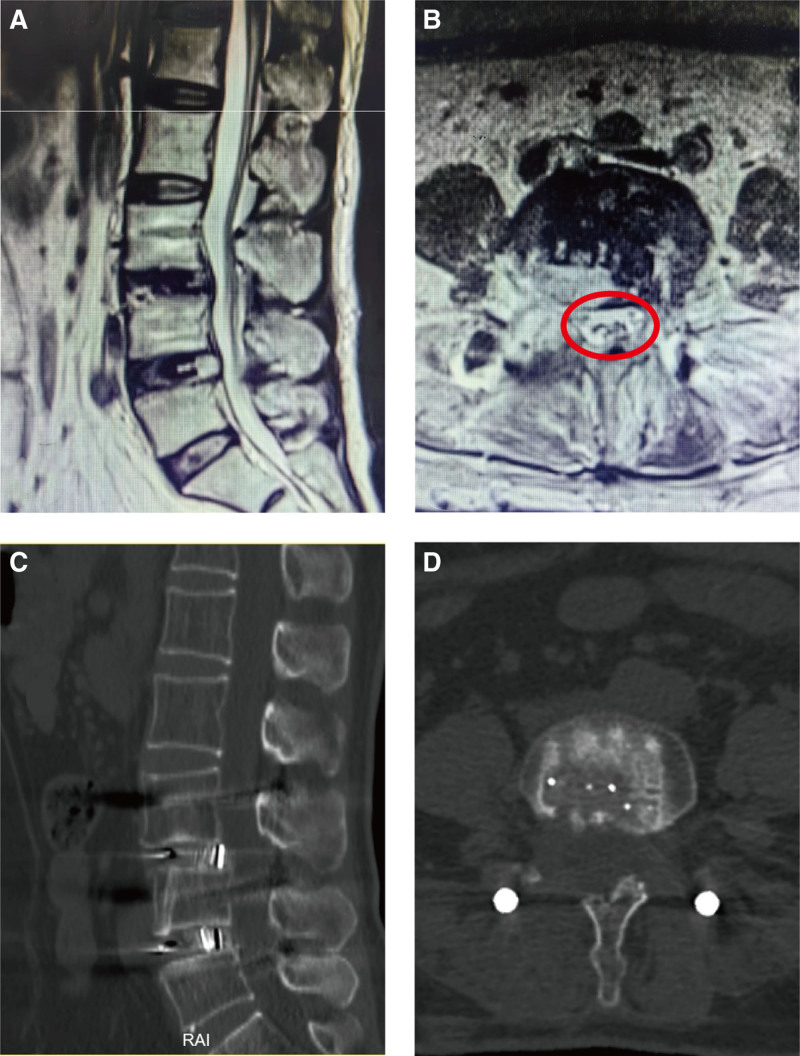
MRI of the lumbar spine for postoperative review at 3 days post-surgery (A, B). CT scan of the lumbar spine for the 1-year postoperative follow-up (C, D). CT = computed tomography, MRI = magnetic resonance imaging.
